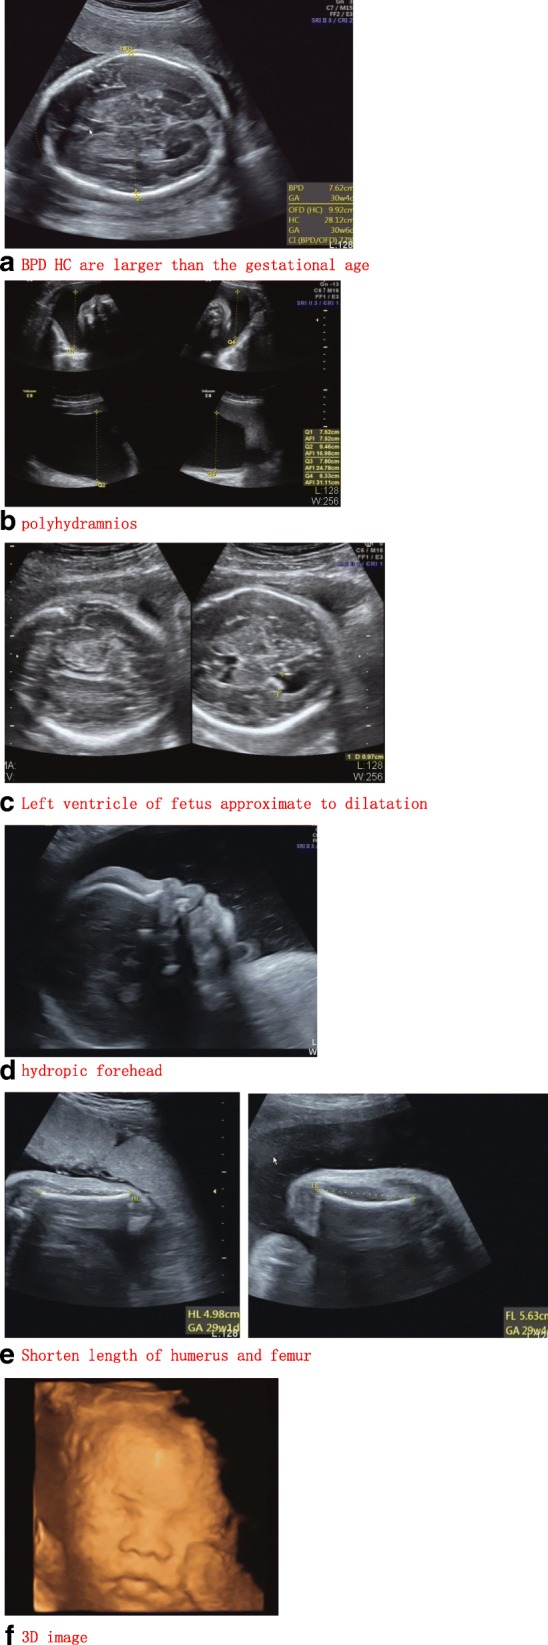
Ultrasound image of 4 fetuses with PKS. a: 2 of 4 fetuses’ biparietal diameter and head circumference were large for gestational age and (b) polyhydramnios; c: Besides mild polyhydramnios, fetus2’s left ventricle approximate to dilatation. d: Fetus4 has hydropic forehead and (e) shorten length of humerus and femur. f: There are no sinificant positive characteristics in all the fetuses’profiles of 3D ultrasound images
